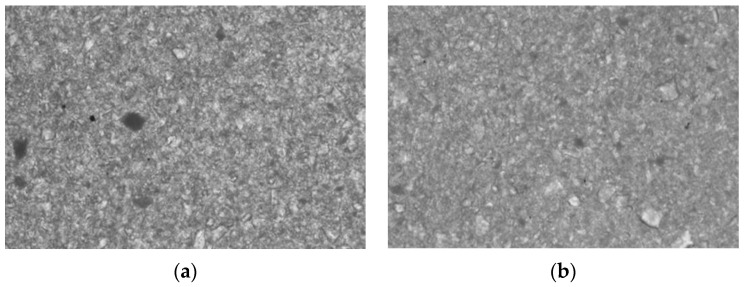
Aspect of ceramic suspensions after solubilization in PBS using ultrasounds: (a) Group 5 (GC) and (b) Group 6 (BC). The photos were taken using a 20× lens.
